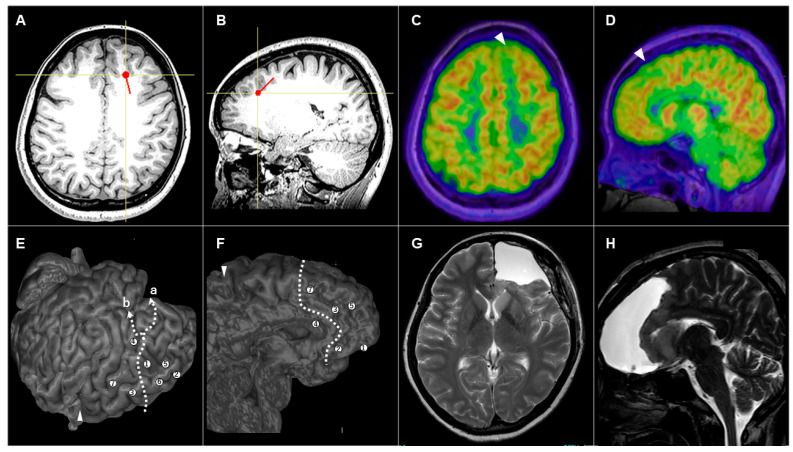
(A,B) Preoperative axial (A) and sagittal (B) magnetic resonance imaging (MRI) showing no apparent abnormalities. The red dot and line depict the estimated dipole in magnetoencephalography. (C,D) Preoperative axial (C) and sagittal (D) 18F-fluorodeoxyglucose positron emission tomography showing a slightly low accumulation in the left superior frontal gyrus (white arrowhead). (E,F) Lateral view (E) and medial view (F) of 3D reconstruction MRI showing the locations of seven SEEG electrodes and resection lines. The posterior margin of resection could not be decided (a or b in (E)). (G,H) Postoperative axial (G) and sagittal (H) MRI showing the resected area.
